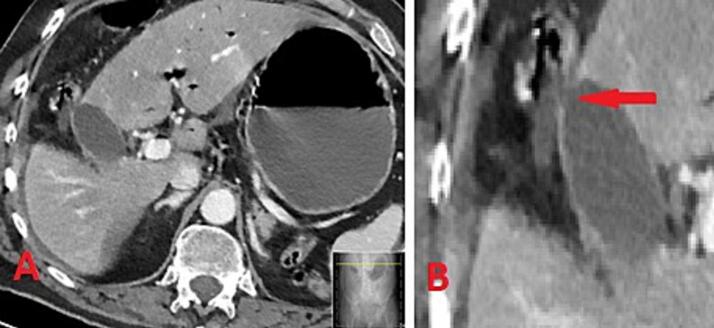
Computed tomography with the abdomen (day 2): A. Frontal view; B. Magnification of the image with an arrow showing perforation (verified after retrospective scrutinization after surgery).
